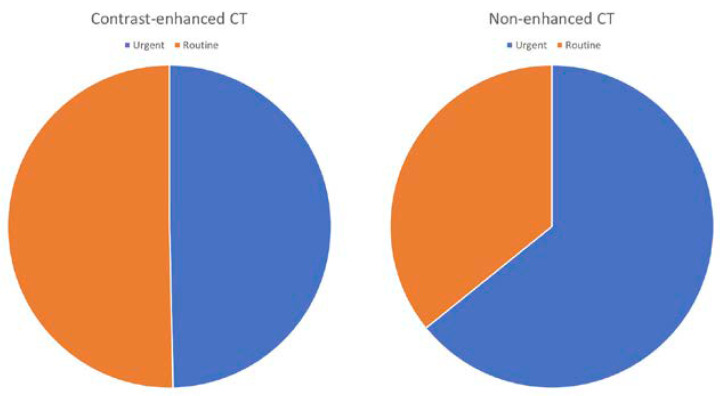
The figure shows the quantitative share of computed tomographies performed urgently (blue) and scheduled (orange). The graph on the right represents tomography performed with contrast enhancement (52.5% urgent), the one on the left without enhancement (35.8% urgent).
